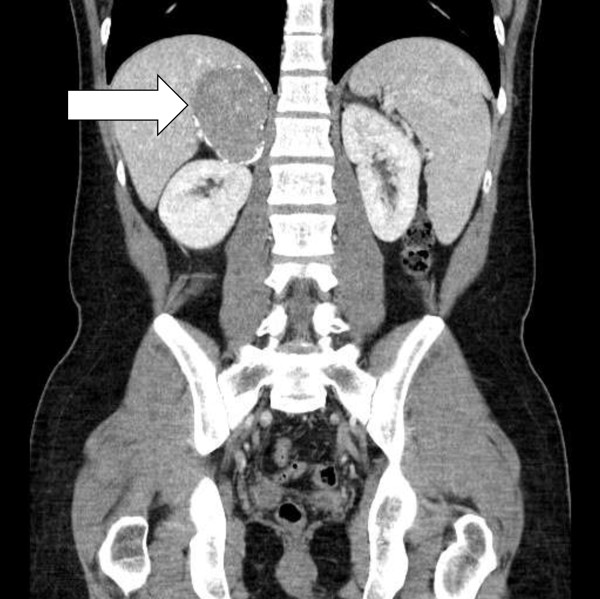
CT image right adrenal gland CFT.
